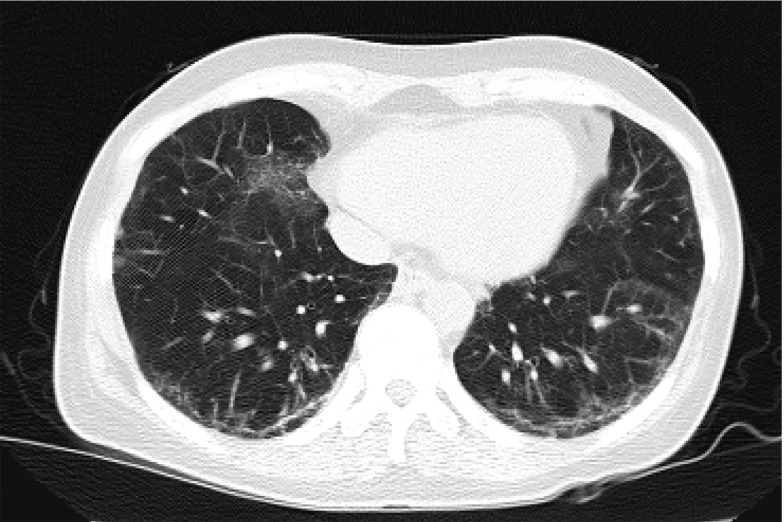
Computed tomography (CT) scan of the lungs showing diffuse reticular opacities and traction bronchiectasis characteristic of pulmonary fibrosis
